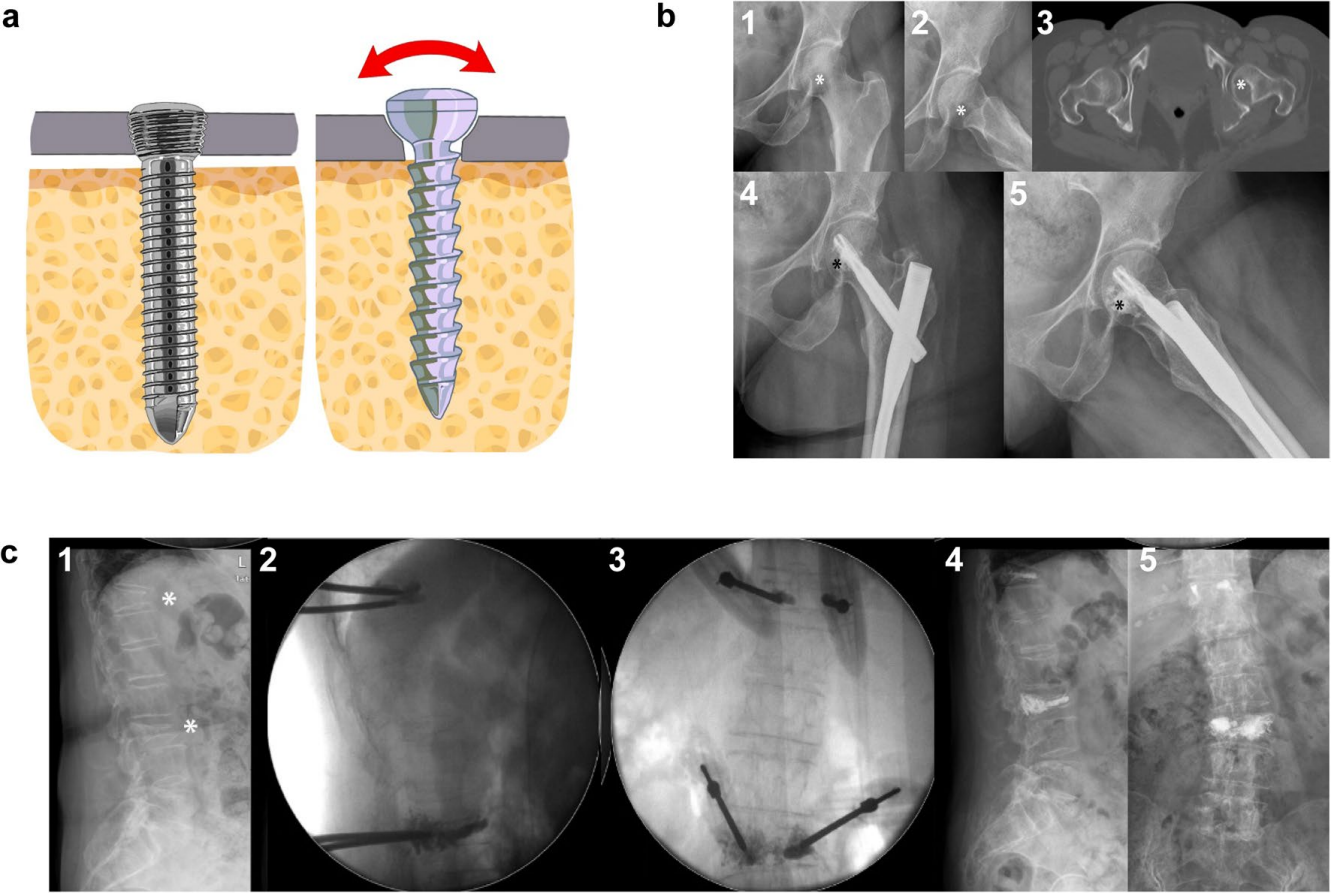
Augmentation technique. A Angular stable locking screw and conventional screw. The threaded screw head locks in the plate hole, providing angular stability and reducing shearing (red arrow). This stability reduces the dependence on the bone–plate interface for stability, protecting periosteal tissue; B Lytic metastatic lesion (white asterisk) of the postero-inferior aspect of the femoral head in a patient with metastatic renal cancer: [1] AP and [2] axial view in conventional radiographs; [3] axial computed tomography; [4] AP and [5] axial view following internal fixation using a cephalomedullary nail with cement augmentation. Note the filling of the lytic lesion in the femoral head (black asterisk). C Fracture of the 11th dorsal and 3rd lumbar vertebra (asterisks) in a patient with multiple myeloma [1]; lateral [2] and anteroposterior [3] intraoperative fluoroscopy of balloon kyphoplasty of the affected vertebrae; lateral [4] and anteroposterior postoperative radiographs [5]. Clinical case courtesy of Dr. Rodrigo Merino, Orthopedic Department, Hospital Universitario 12 de Octubre, Madrid
